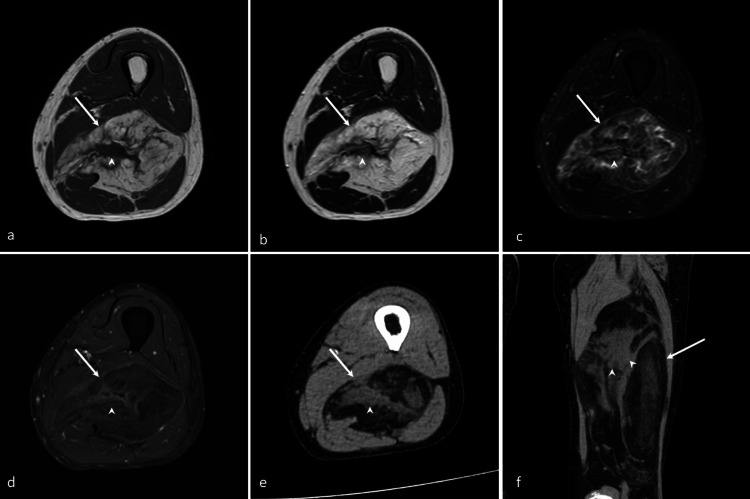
A 77-year-old woman with an ASCPLT in the thigh Axial T1WI (a) and axial T2WI (b) show well-defined intermuscular fatty mass (arrows) containing non-fatty components (arrowhead). Axial FST2WI (c) and axial FSCET1WI (d) demonstrate low signal intensity and mild enhancement of non-fatty components, respectively. Axial CT (e) and coronal CT (f) show a well-defined intermuscular fatty mass (arrow) with non-fatty components (arrowhead). The imaging findings are consistent with a spindle cell lipoma pattern.
